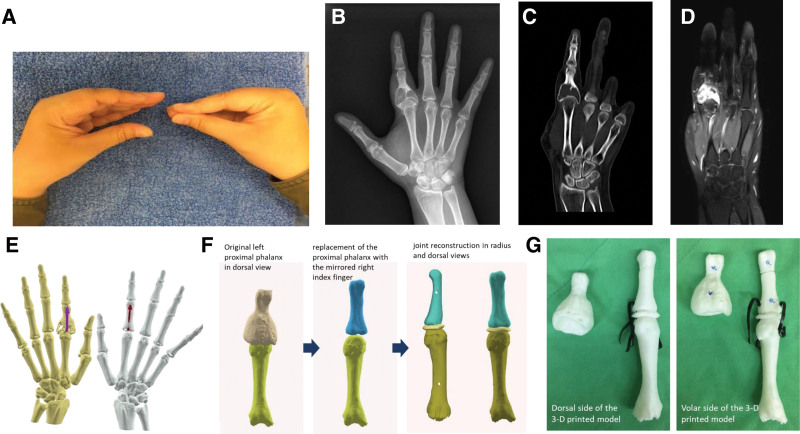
Preoperative images: (A) the limited ROM of the left MCP joint before surgery; (B) X-ray shows a radiolucent lesion with a thinning cortex; (C) CT reveals an intact bony structure but a thinning cortex and no joint space involvement; (D) MRI-T2 weight indicates no soft tissue involvement; (E) 3D lesions compared with a typical presentation; (F) procedures for reconstruction; and (G) Dorsal and volar views of the 3D printed model. 3D = three-dimensional, CT = computed tomography, MCP = metacarpophalangeal, MRI = magnetic resonance imaging, ROM = range of motion.
